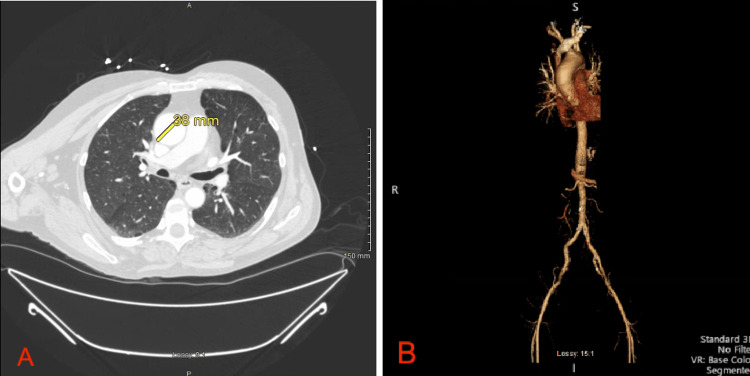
A: Axial section of a computed tomography angiogram of the chest demonstrating emphysematous lung parenchymal changes and an ectatic ascending aorta measuring 3.8 cm. B: Three-dimensional reconstruction of the chest, abdomen, and pelvic arterial vasculature. cm: centimeter; mm: millimeter
